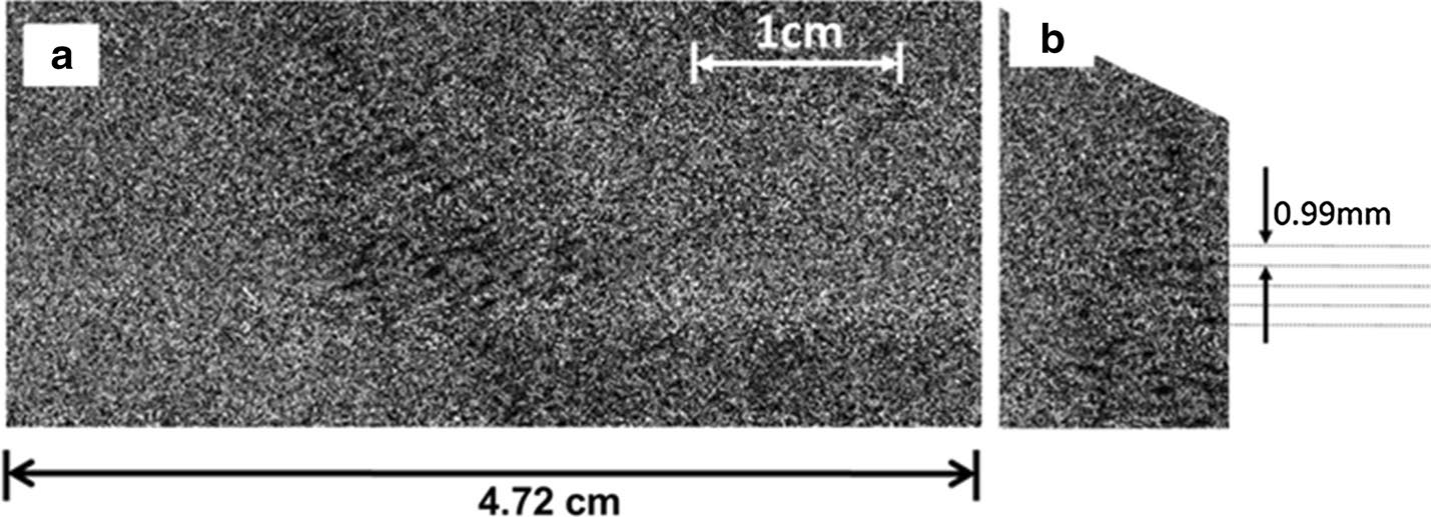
Vertical* scan of the whole ultrasound beam of a therapeutic ultrasound system below the tip of the probe at 0.8835 MHz and 14 W (a). The sequence form of cavitation bubbles’ location with approximately same intervals between sequences (b)
